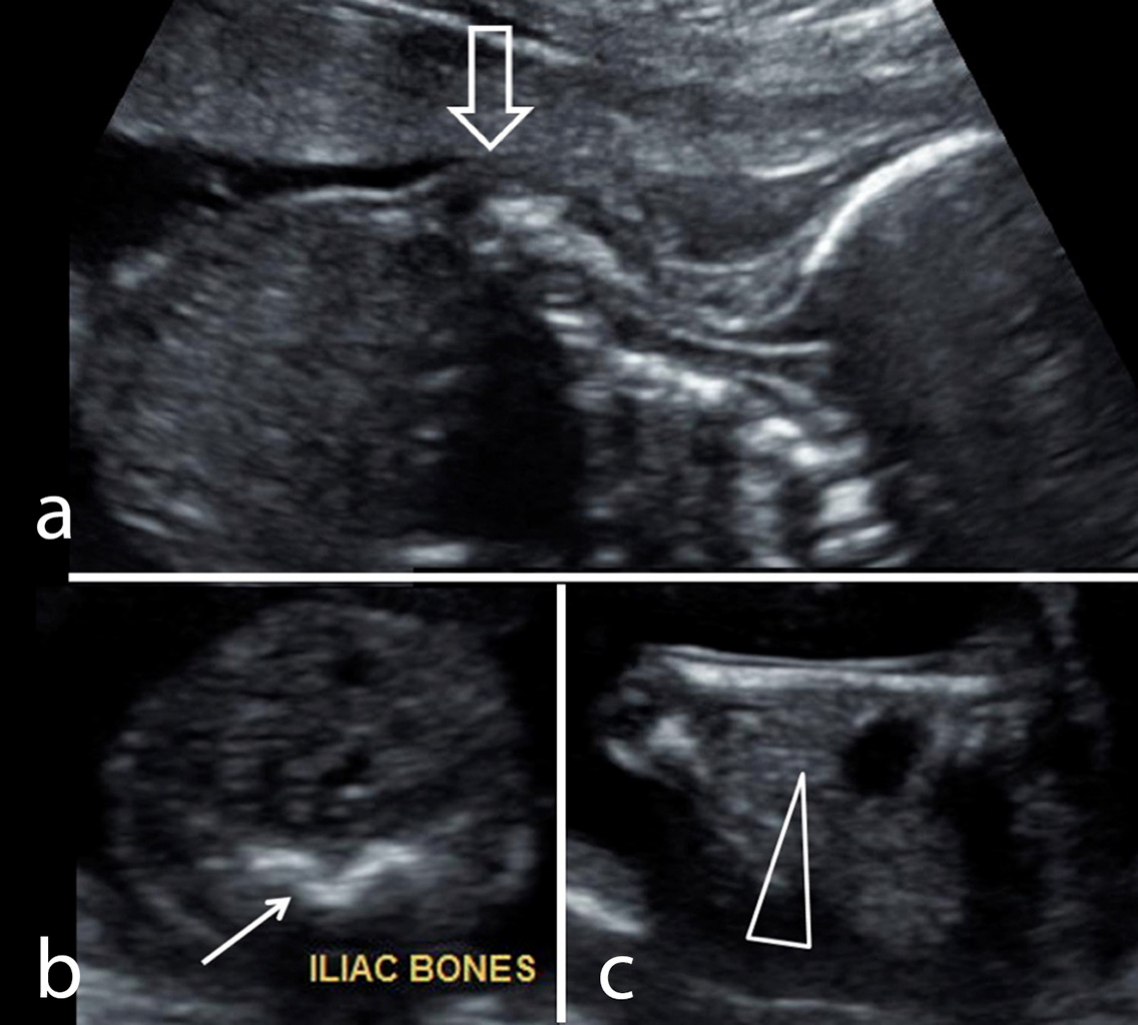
(a) Longitudinal sonogram of the foetus demonstrate a local hump in the dorsal region (open arrow) at the site of termination of the spinal column. No osseous elements are noted distally. (b) Transverse scan at the pelvic level shows fusion of both iliac bones in the midline, showing a “shield-like” configuration (arrow). (c) Image of the knee shows flexed position of the knee joint with a popliteal pterigium (arrow head). There is polyhydramnios.
